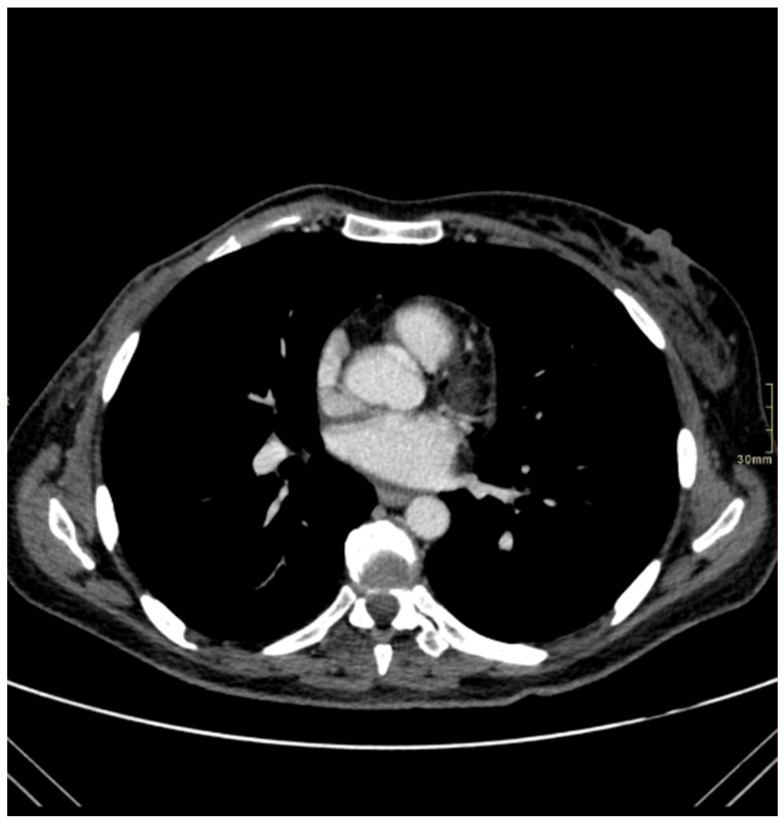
Absence of radiological evidence of neoplastic recurrence in the 2 months follow-up whole-body CT scan.
